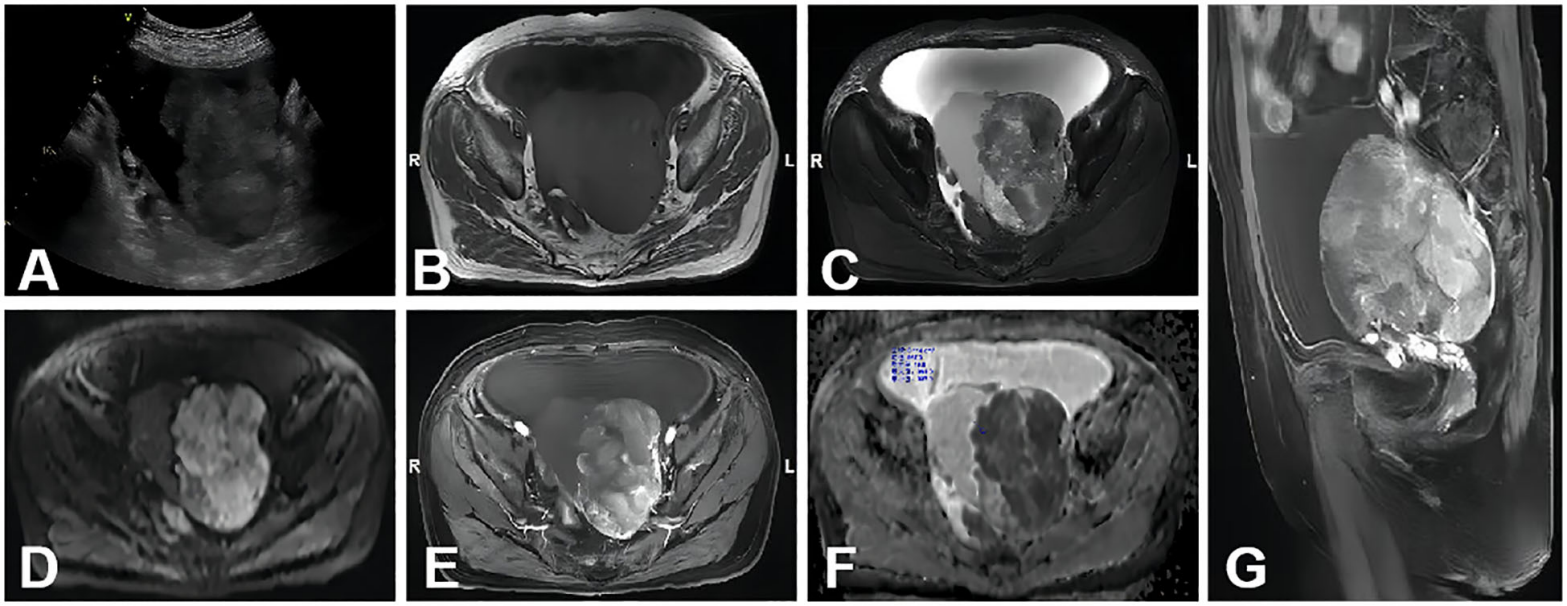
A 70-year-old woman with stage I CCC on the left ovary. (A) A non-homogeneous middle-hypoechoic solid mass was detected by ultrasound examination. (B–D) A lobulated cystic-solid mass with hemorrhage signal. The large solid portion showed mainly isointense, hyperintense, and hyperintense signal intensities on axial T1WI, T2WI, and DWI (b=1,000 s/mm^2^), respectively. (E) Axial contrast-enhanced T1WI showed that the solid portion was of intensive enhancement. (F) On the ADC map, the mean ADC value was 0.719 × 10^−3^ mm^2^/s. (G) Sagittal contrast enhanced T1WI showed the mass was similar to (E).
